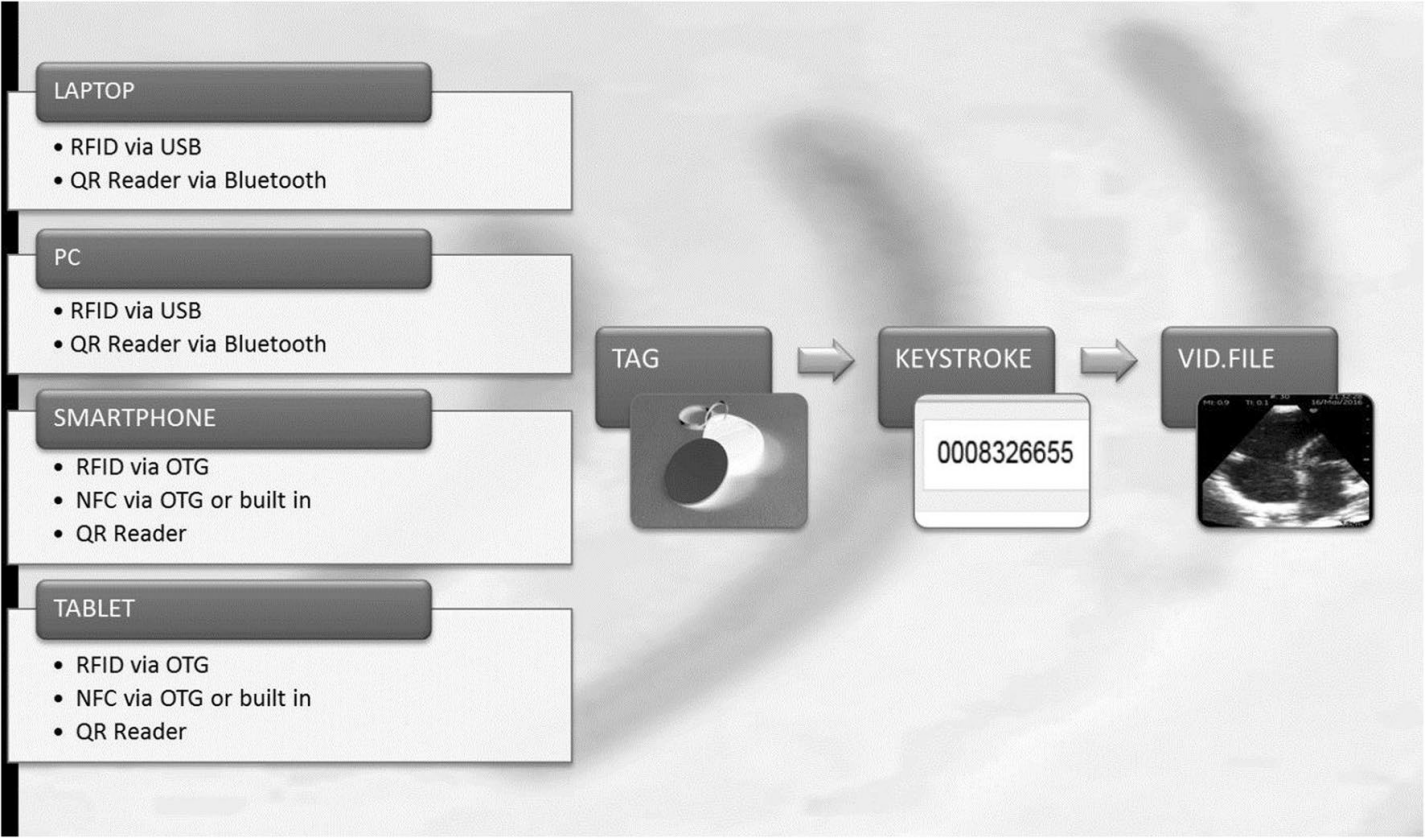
Different devices such as laptop computers, desktop computers/PCs, smartphones as well as tablets can be used to display video files, that is, ultrasound loops, respectively. These are triggered by tags, the content of which is read as a keystroke. *RFID* radio-frequency identification, *USB* universal serial bus, *QR* quick response, *OTG* on the go, *NFC* near-field communication. VID. FILE video of ultrasound loop stored as a separate file
